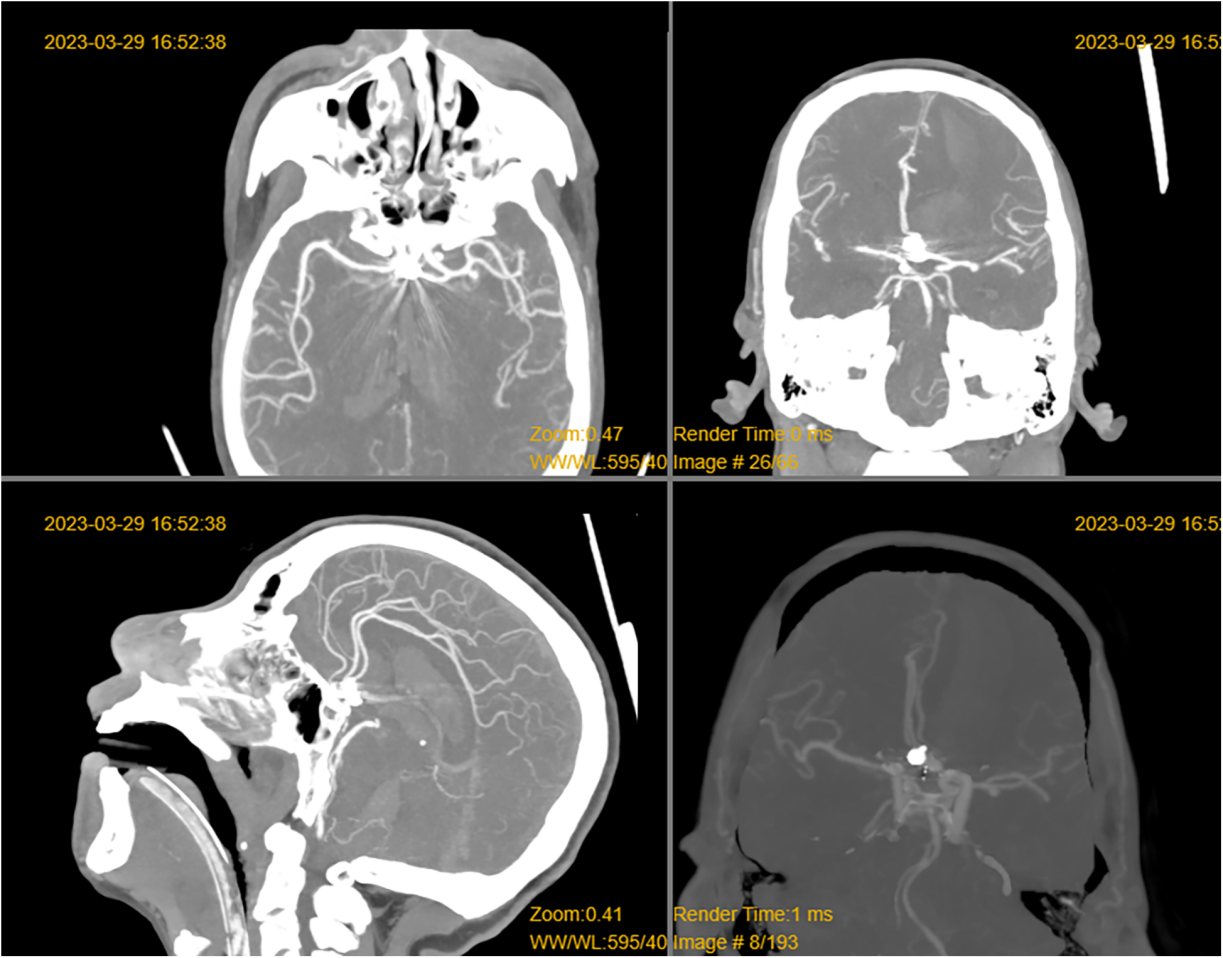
Horizontal, coronal, and sagittal reconstructions of the CTA examination.
